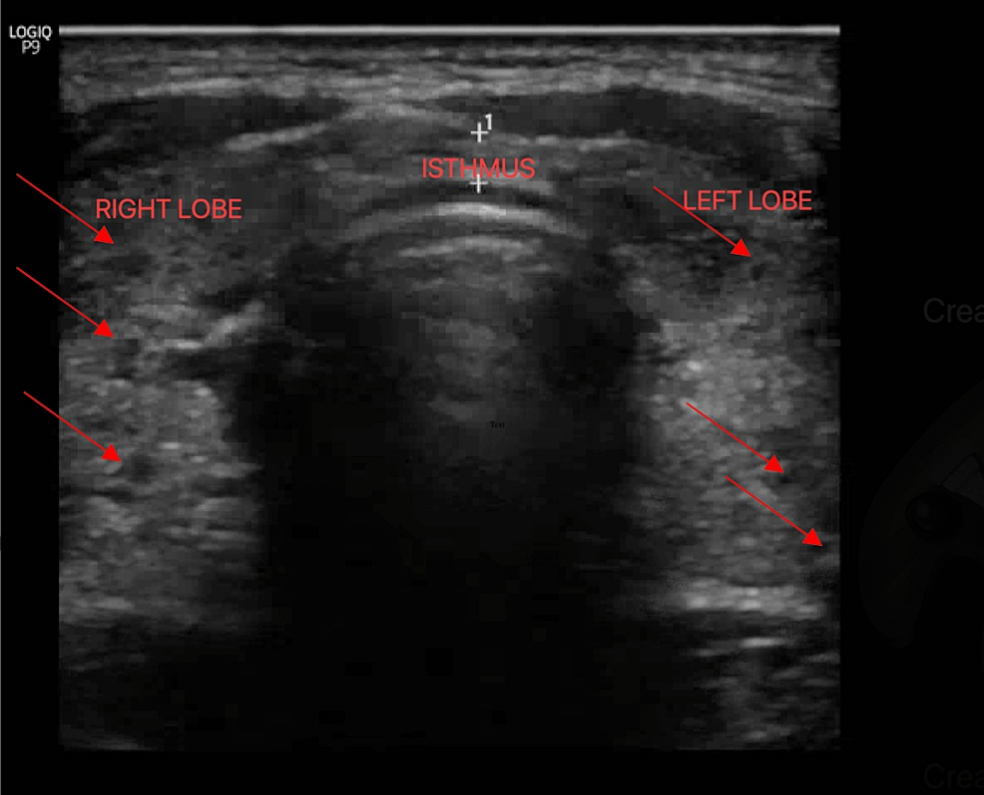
Ultrasound of the thyroid gland showing enlarged right and left lobes with arrows representing bilateral sub-centimeter thyroid nodules
